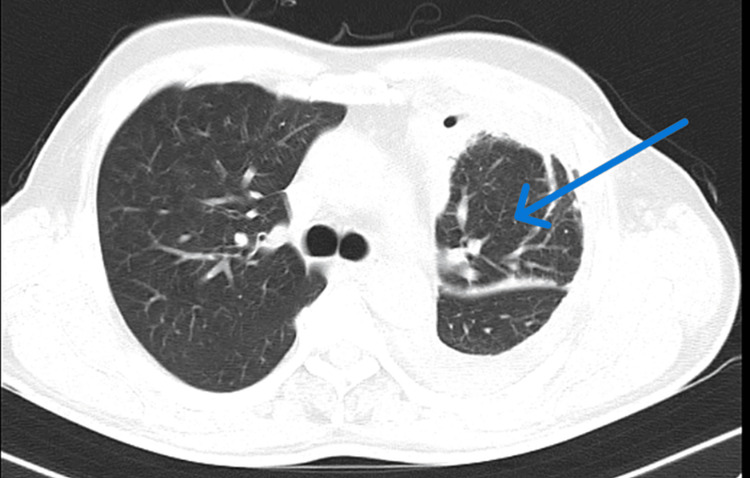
CT thorax of the chest; lung window showing trapped lung (blue arrow) CT: computed tomography
